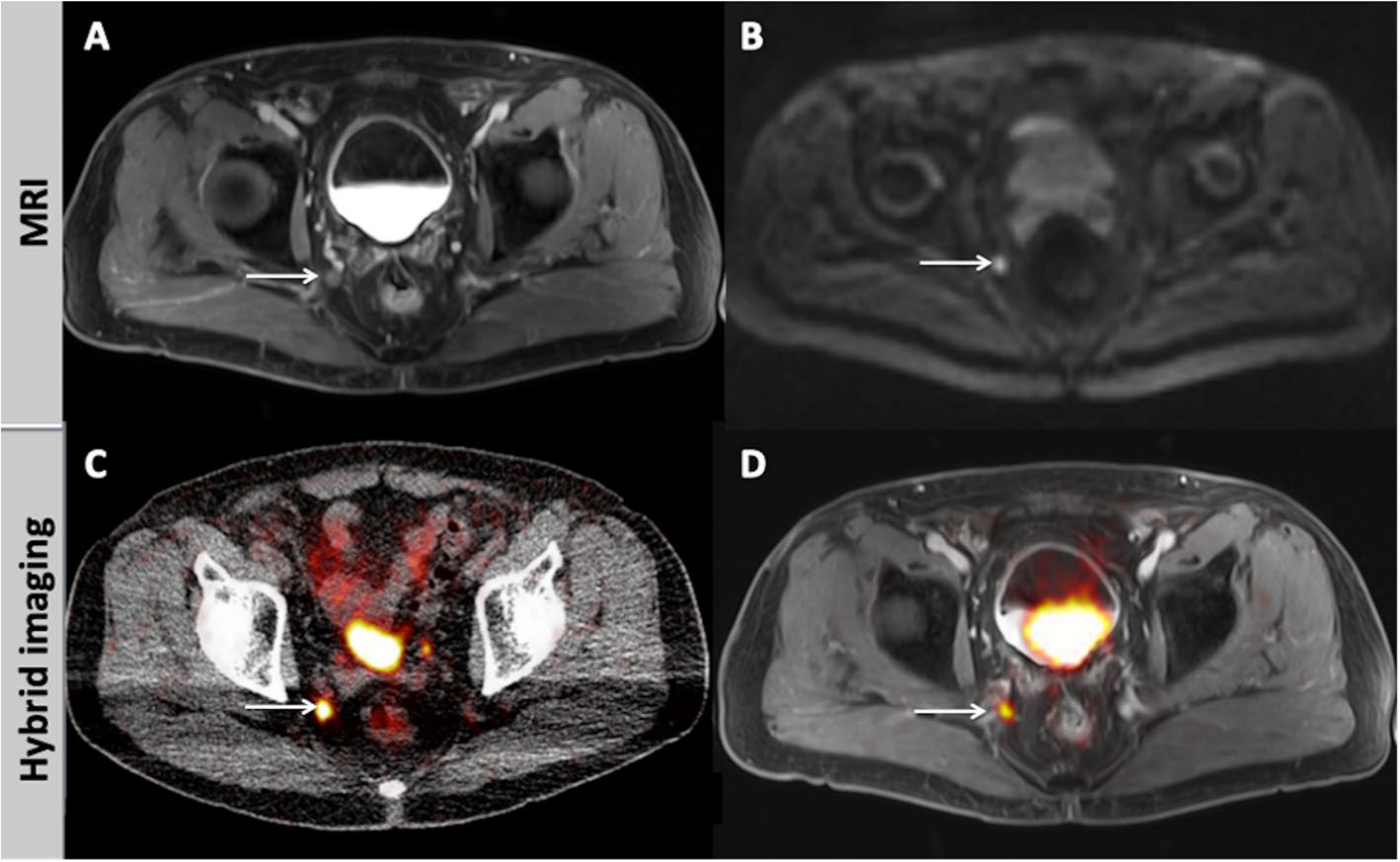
A 75-year old patient with biochemical recurrence of PCa referred to our institution for imaging. His PSA level at the time of examination was 4.1 ng/ml. Contrast enhanced-T1w fatsat (A) and DWI (b800; B) at the pelvic level show a 9 mm, rounded, right obturator lymph node with irregularly spiculated border and heterogeneous contrast agent enhancement (arrow, A) and distinct diffusion restriction with signal increase in the b800 image (arrow, B). The lymph node also exhibited signal decrease in the corresponding ADC map (not shown). In the fused hybrid modalities PET-CT (C) and PET-MRI (D), the lymph node also shows a focal, intensive [^68^Ga]PSMA-11 uptake, suggestive of metastatic involvement (arrow)
